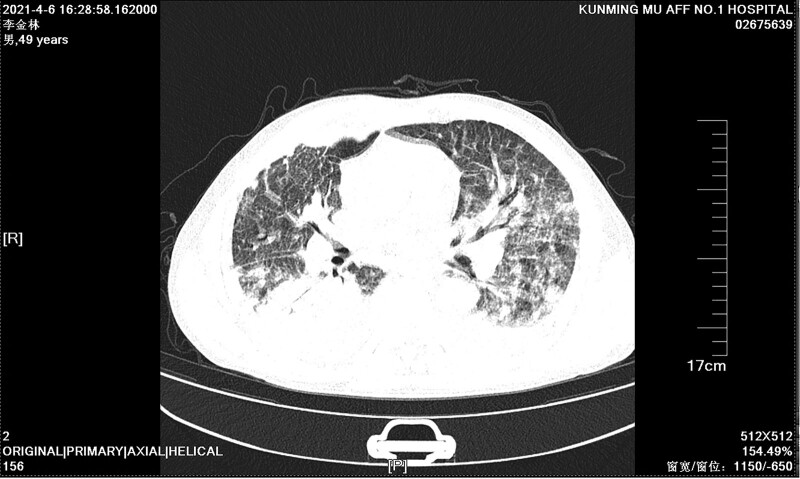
Chest CT on April 6, 2021 showed that the upper and lower lobes of the right lung had larger flaky consolidation, with bronchial air signs inside, multiple high-density nodules of varying sizes in both lungs, and thickened upper lobular lobules in both lungs. The exudation increased, and the mediastinum still has no clear enlarged lymph node shadow. CT = computed tomography.
